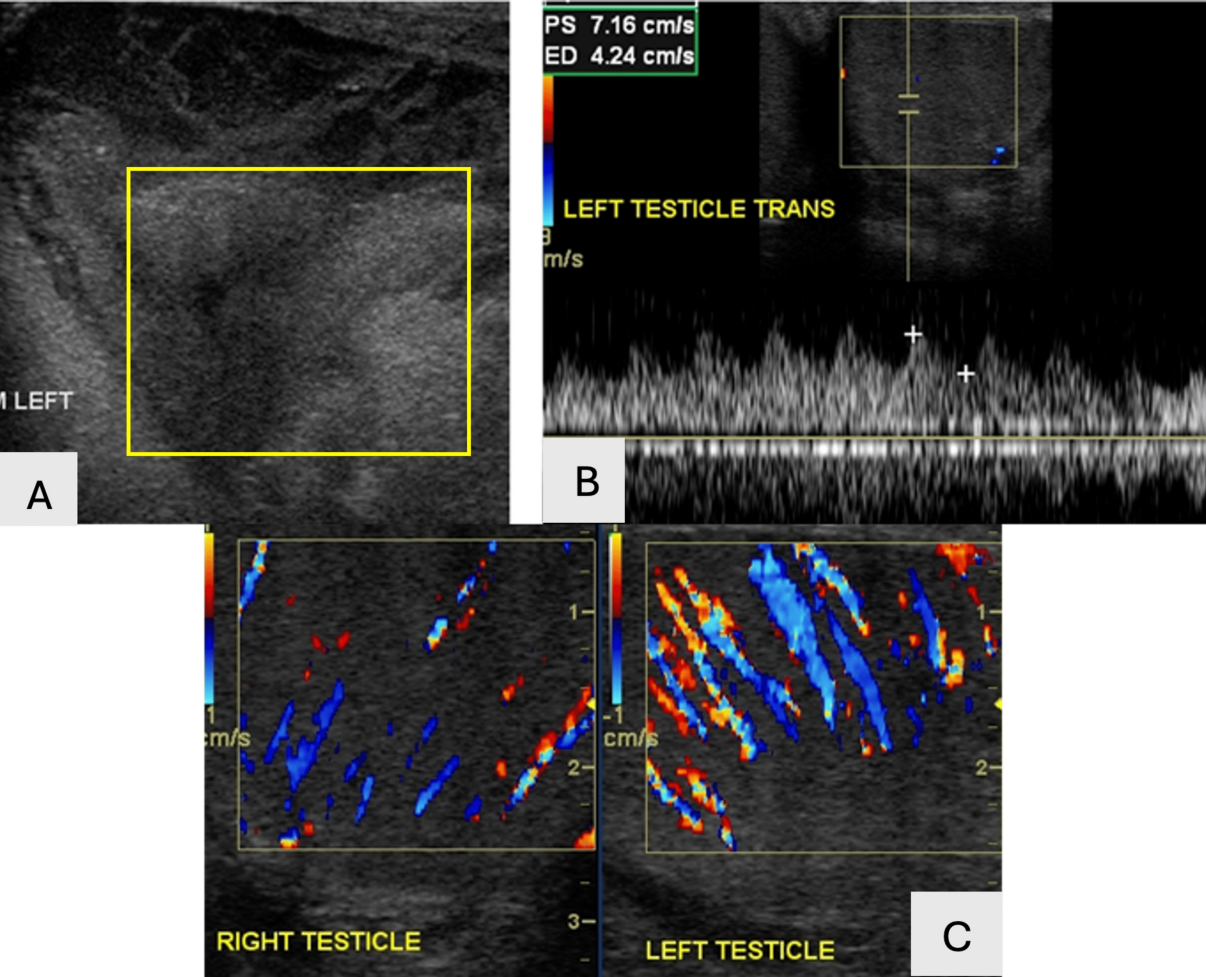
Grayscale (18A) (yellow rectangle) and color Doppler (18B and 18C) US images demonstrate left epididymal head enlargement with decreased vascular resistance and increased diastolic flow on spectral waveform.
